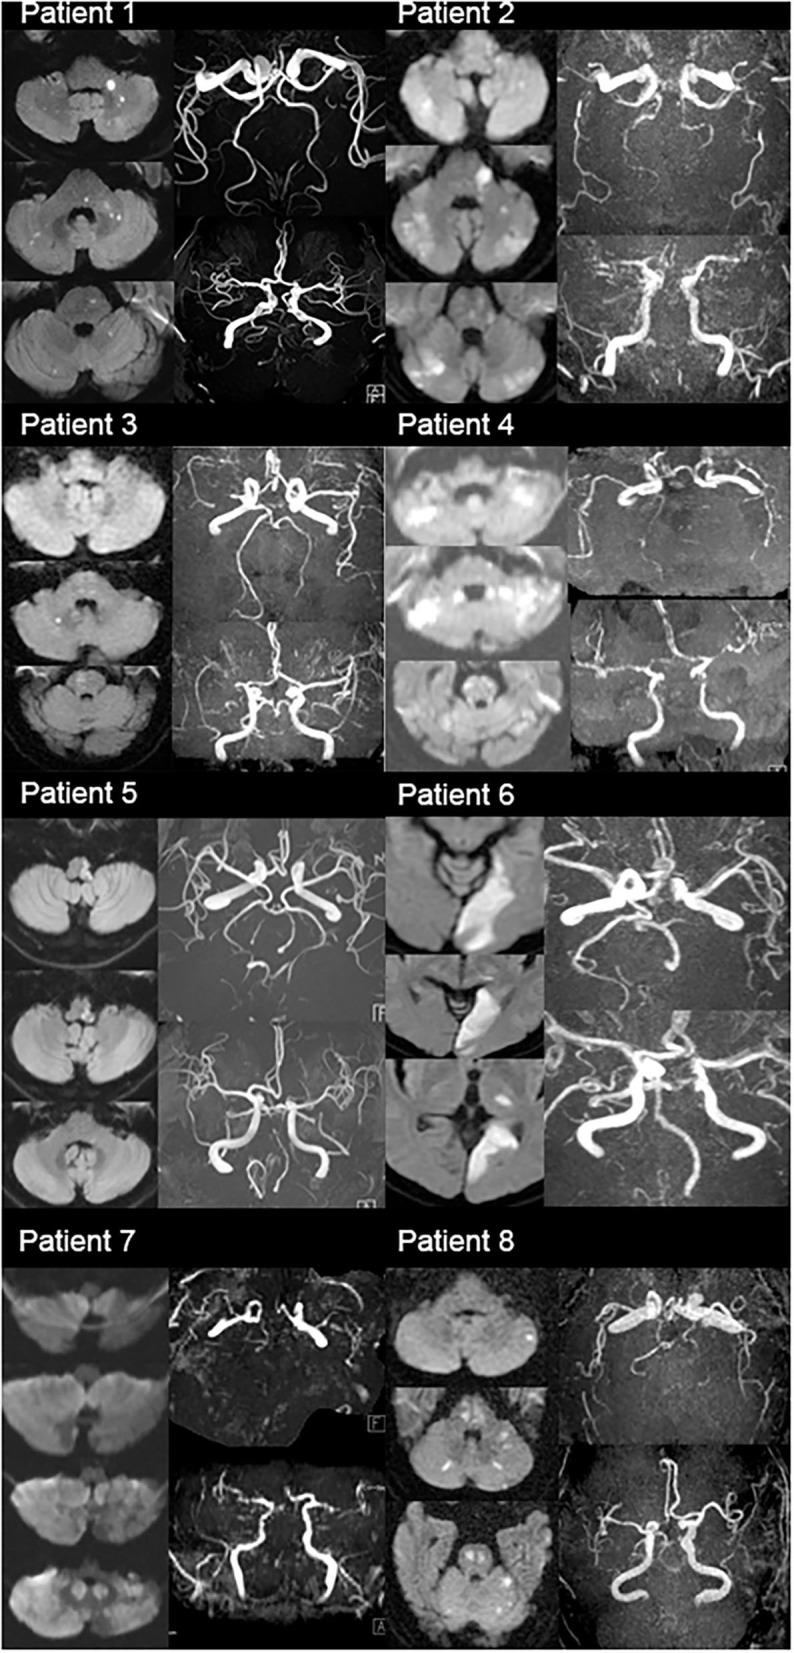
MRI diffusion-weighted images (DMI) and MRA images of total eight patients (MRI DWI showed multiple infarctions in these patients; MRA showed fetal type PCA, vertebral hypoplasia, small caliber of basilar artery, or invisible vertebrobasilar arteries).
